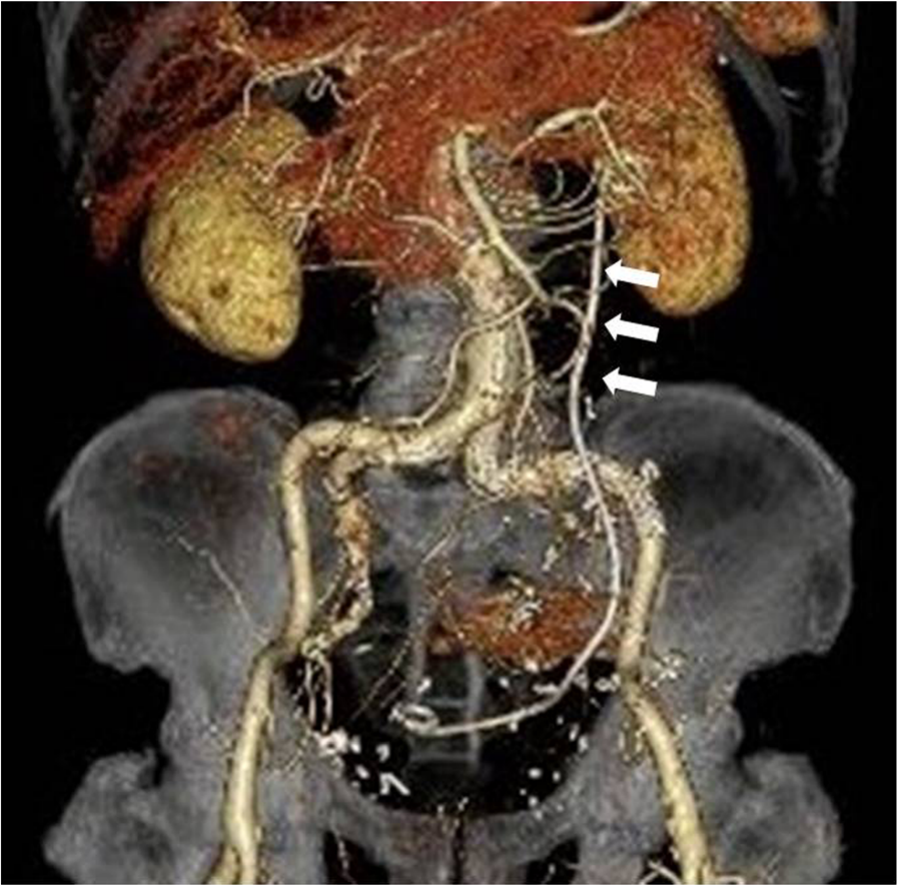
Postoperative computed tomography demonstrates endovascular stent grafting from the left common iliac artery to the left external iliac artery and full occlusion of the internal iliac aneurysm. Arrows indicate the ureteral stent
